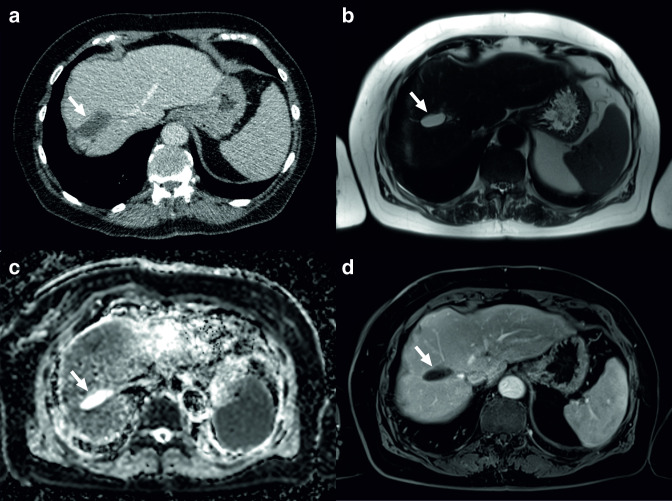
A 72-year-old female underwent CT of the chest because of respiratory symptoms. CT was negative for intrathoracic pathology, but did show a hypodense lesion of unclear nature in the liver (a, arrow) as an incidental finding. MRI showed the lesion to be hyperintense on *T*
